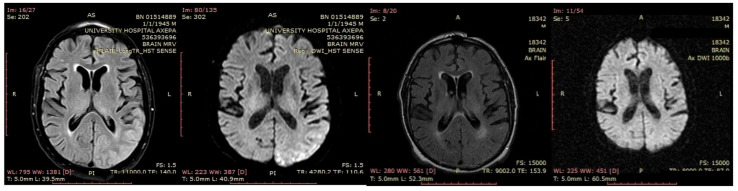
From left to right: the first and the second images demonstrated signal abnormality in the cortex of the left temporal–parietal–occipital areas in fluid-attenuated inversion recovery (FLAIR) and diffusion-weighted imaging (DWI), respectively, which resolved in follow-up MRI (third image—FLAIR, fourth image—DWI).
